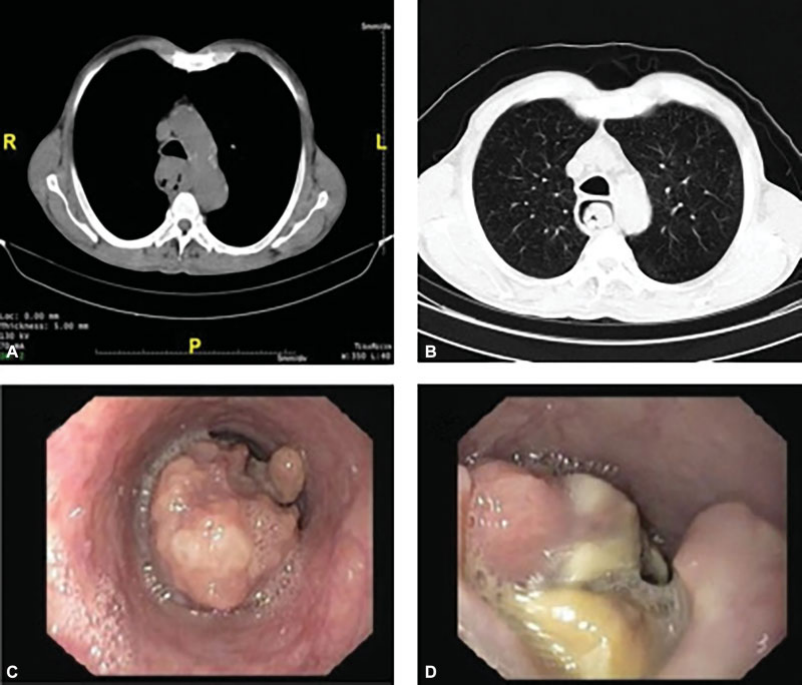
(A,B) Computed tomography images of the tumor. (C,D) Endoscopic images of tumor.
